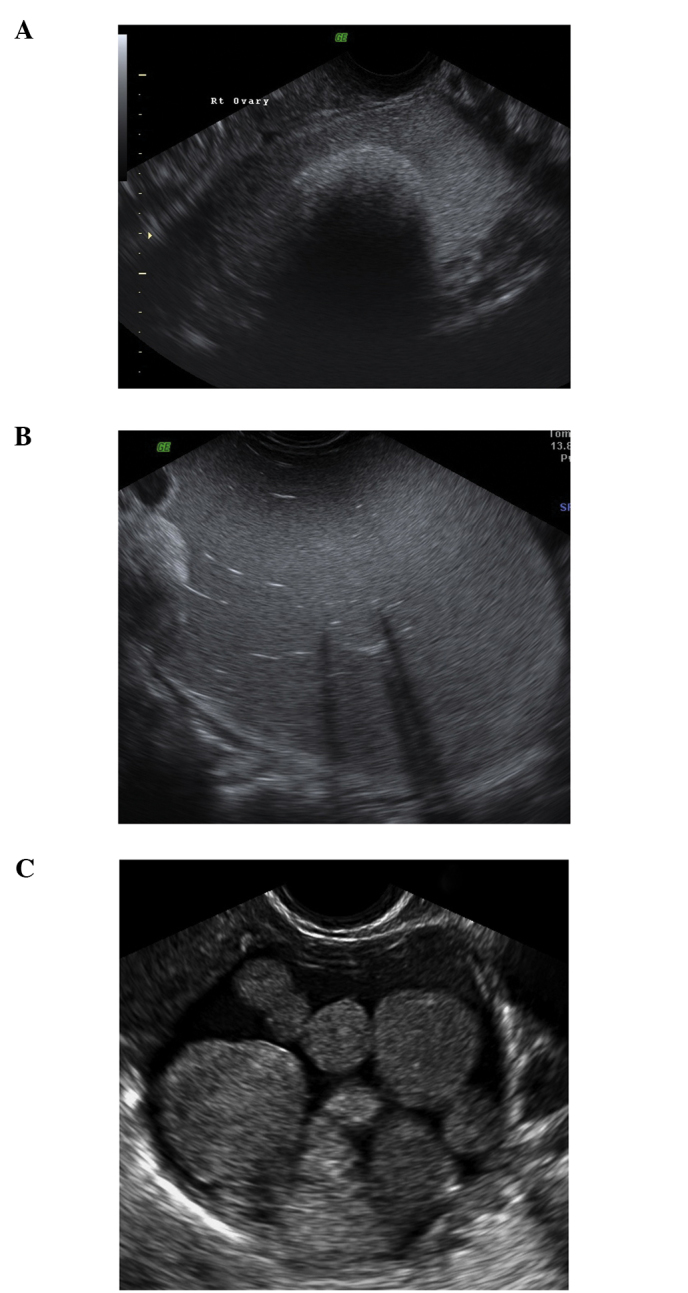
Ultrasound features of dermoid cysts. (A) Rokitansky nodule with a strong acoustic shadow. (B) Acoustic shadows and bright echoes representing hair in the cyst. (C) Unusual but interesting presentation of a dermoid cyst which has been described as ‘floating balls’ - secondary to hyperechoic intracystic fat balls.
